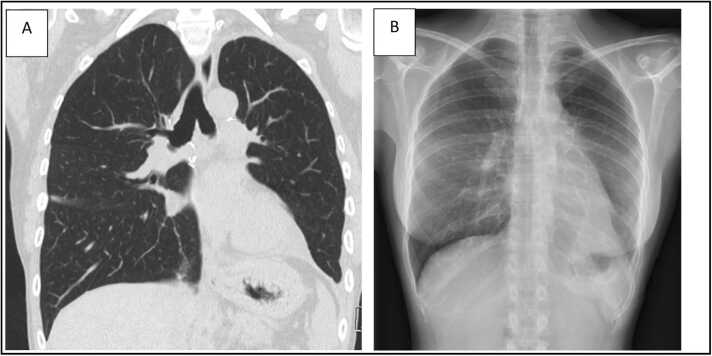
A: Chest computed tomography scan 162 days postoperatively; B: Chest X-ray 222 days postoperatively (superposition of bilateral breast implants).
